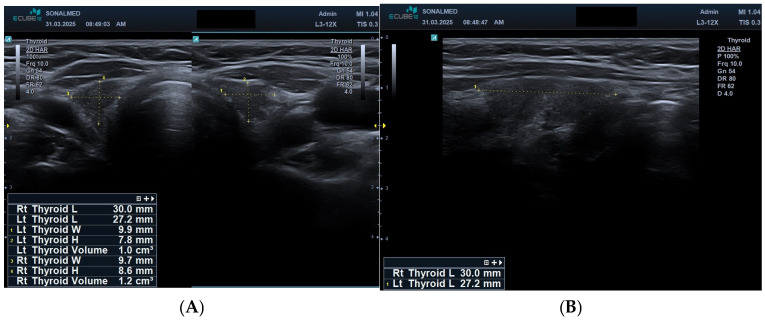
(A) Transverse grey-scale ultrasound image of the thyroid gland after three years of sunitinib therapy. The image shows a markedly reduced thyroid volume (left lobe: 1.0 cm^3^; right lobe: 1.2 cm^3^) with substantial parenchymal loss, decreased echogenicity, and pronounced structural heterogeneity. These findings indicate advanced thyroid atrophy as a consequence of long-term sunitinib exposure. (B) Longitudinal grey-scale ultrasound image of the thyroid gland after three years of sunitinib therapy. The image demonstrates a thin, markedly reduced thyroid lobe with significantly diminished parenchymal volume and decreased echogenicity. The flattened glandular contour reflects advanced thyroid atrophy, consistent with long-term sunitinib-induced structural deterioration.
